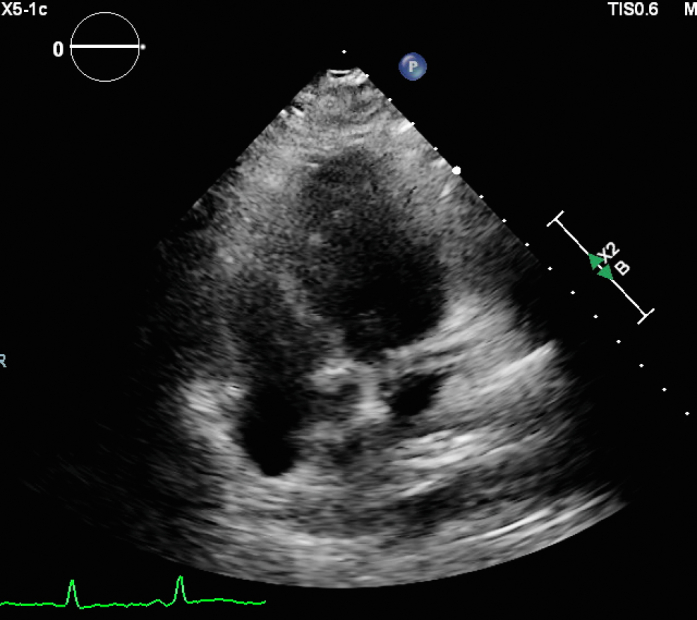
Echocardiogram After Pericardial Drain Removal Demonstrating No Pericardial Effusion
